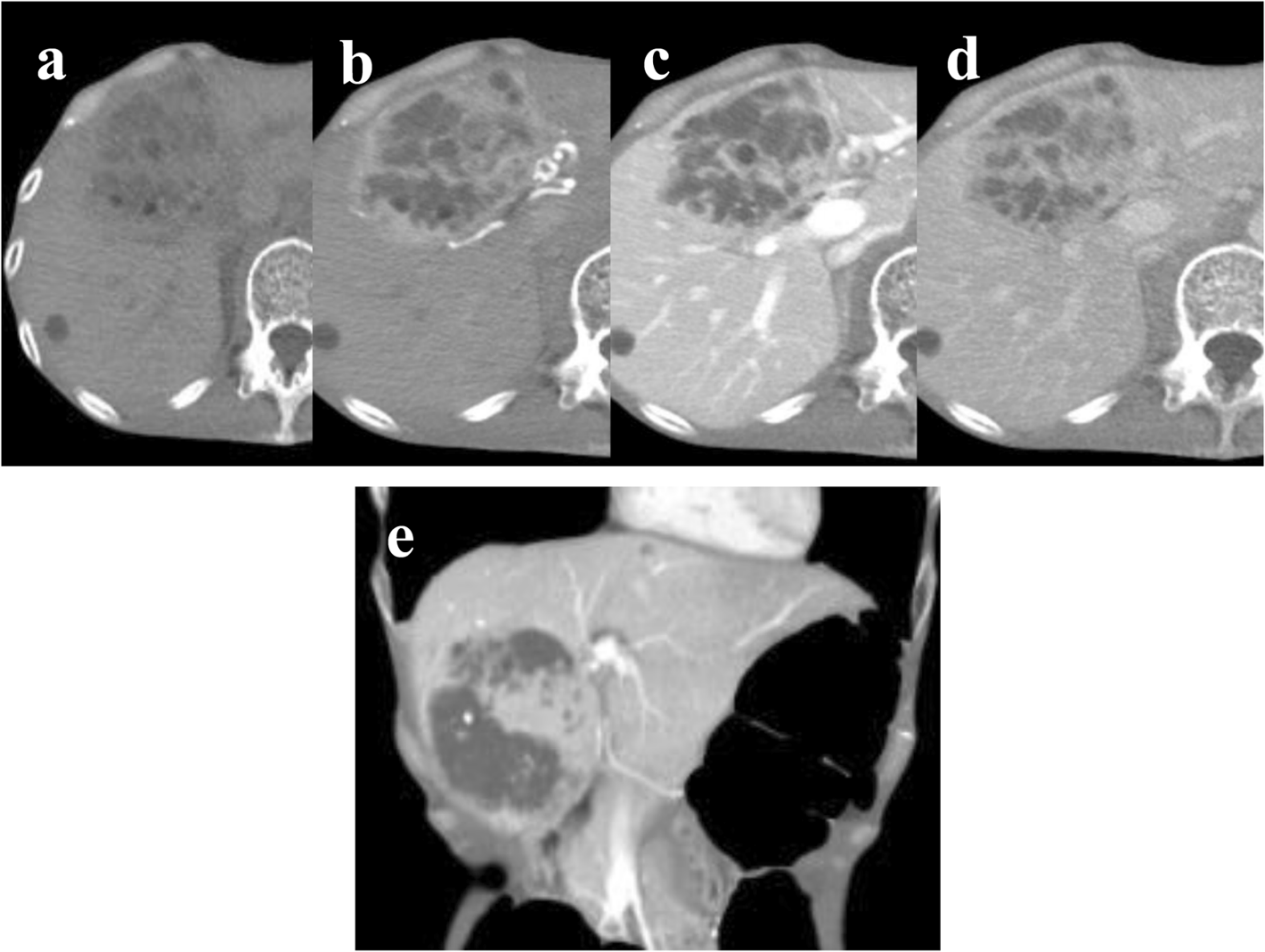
Computed tomography (CT) imaging. a Plain CT showed a slight low-density tumor (diameter, 80 mm) in segments S4 + S5. b Contrast-enhanced CT of the arterial phase showed a hypervascular mass. c Portal phase: the contrasting effect continued. d Delayed phase: the tumor looked similar to a normal liver. e Coronal section in the portal phase: the irregular non-contrasting area was detected inside of the tumor and accompanied by calcification, cystic parts, fatty deposition, and suspected partial hemorrhagic necrosis
